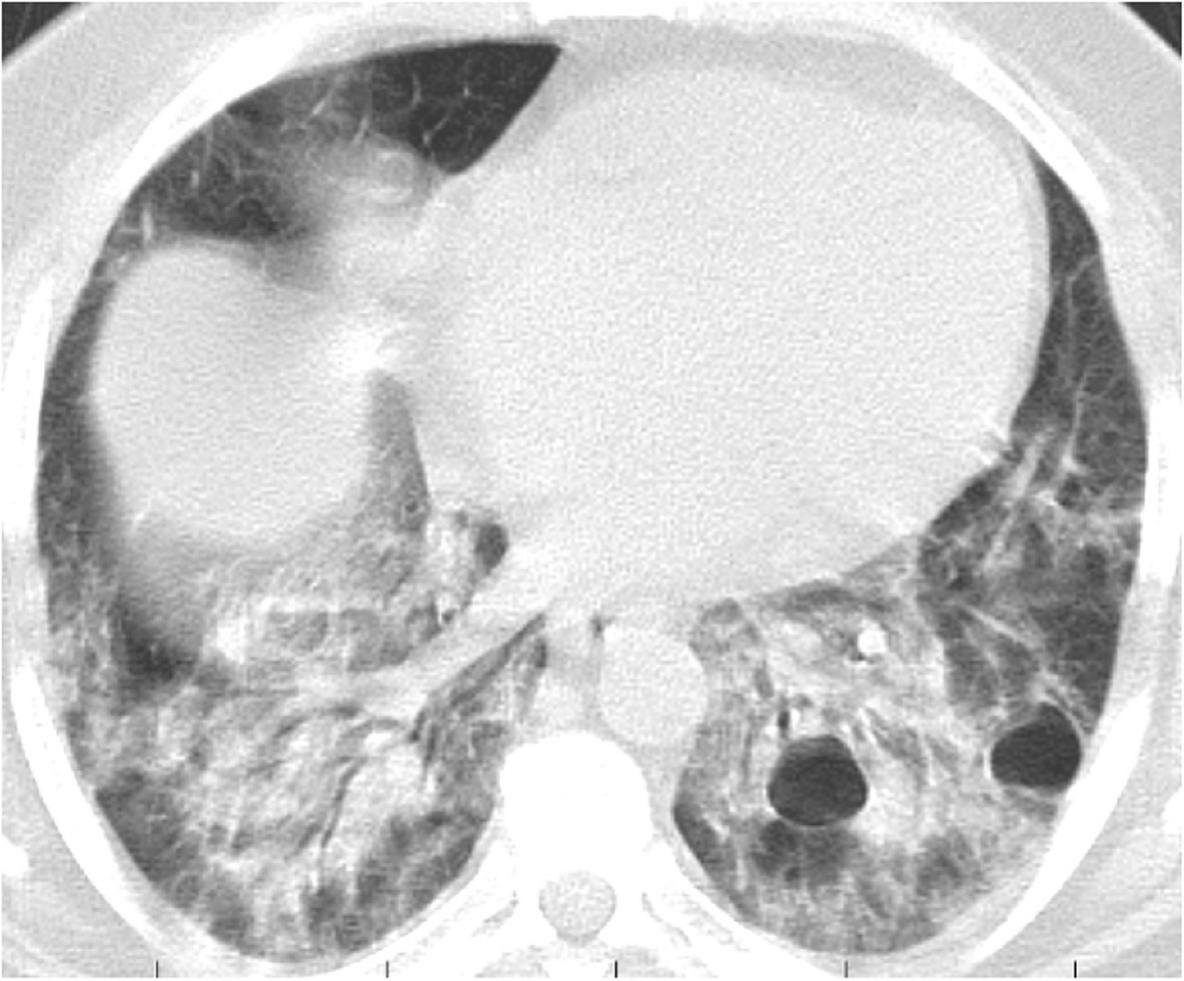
A 50-year-old male patient with COVID-19. The patient is a known hypertensive and diabetic. CT chest axial image shows bilateral extensive consolidation of both lower lobes with air-bronchogram. Two air–containing lungs cysts are seen in the left lower lobe lesion
